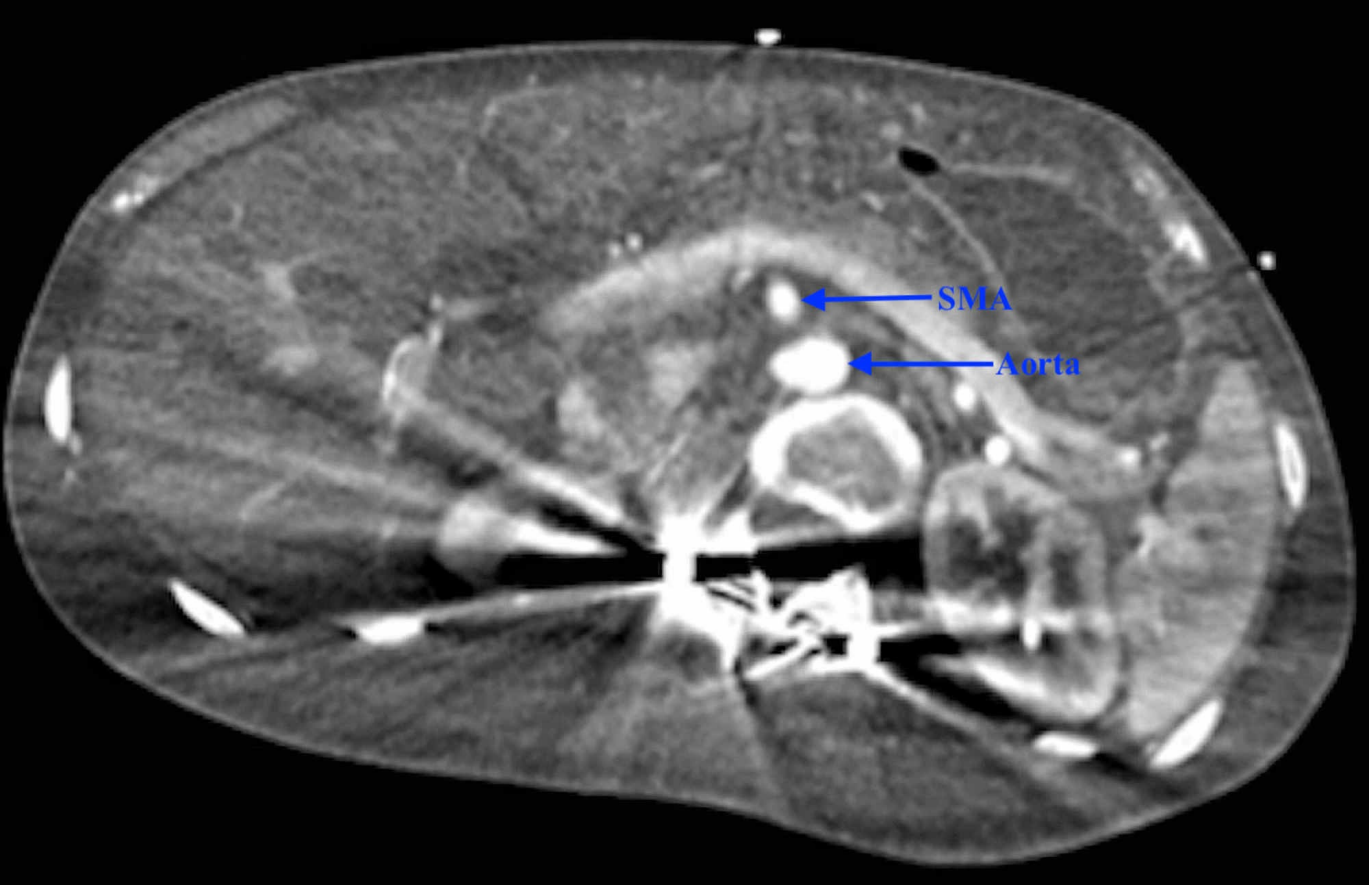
Computed tomography (CT) scan of the abdomen (axial section) with contrast showing resolution of gastric distention with unchanged AMD <1 cm SMA: superior mesenteric artery; AMD: aorto-mesenteric distance.
